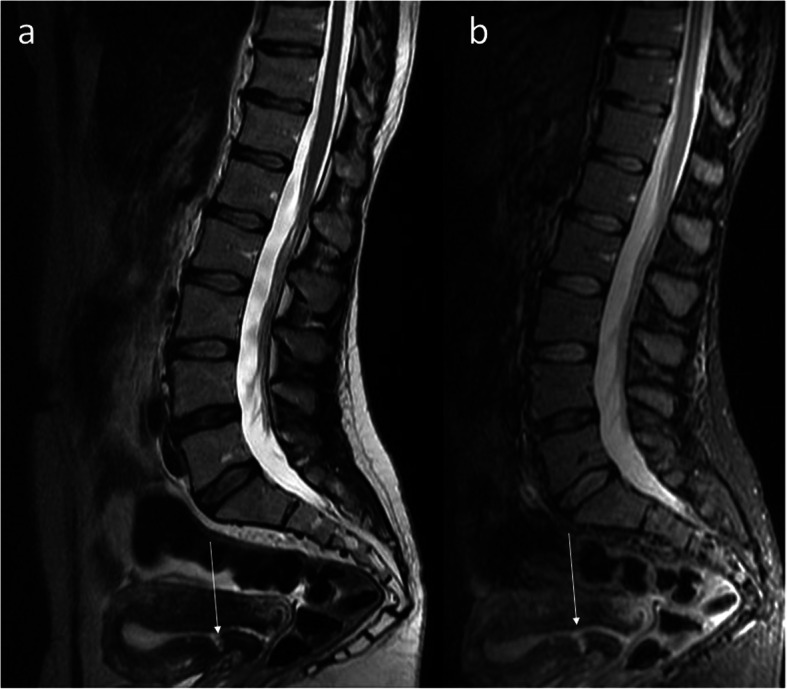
MRI of the lumbar-sacral part of the vertebral column in the sagittal plane. a FSE/T2WI. b STIR image. Post-C-section niche, hyperintense against the background of the hypointense myometrium, is shown by the arrows
